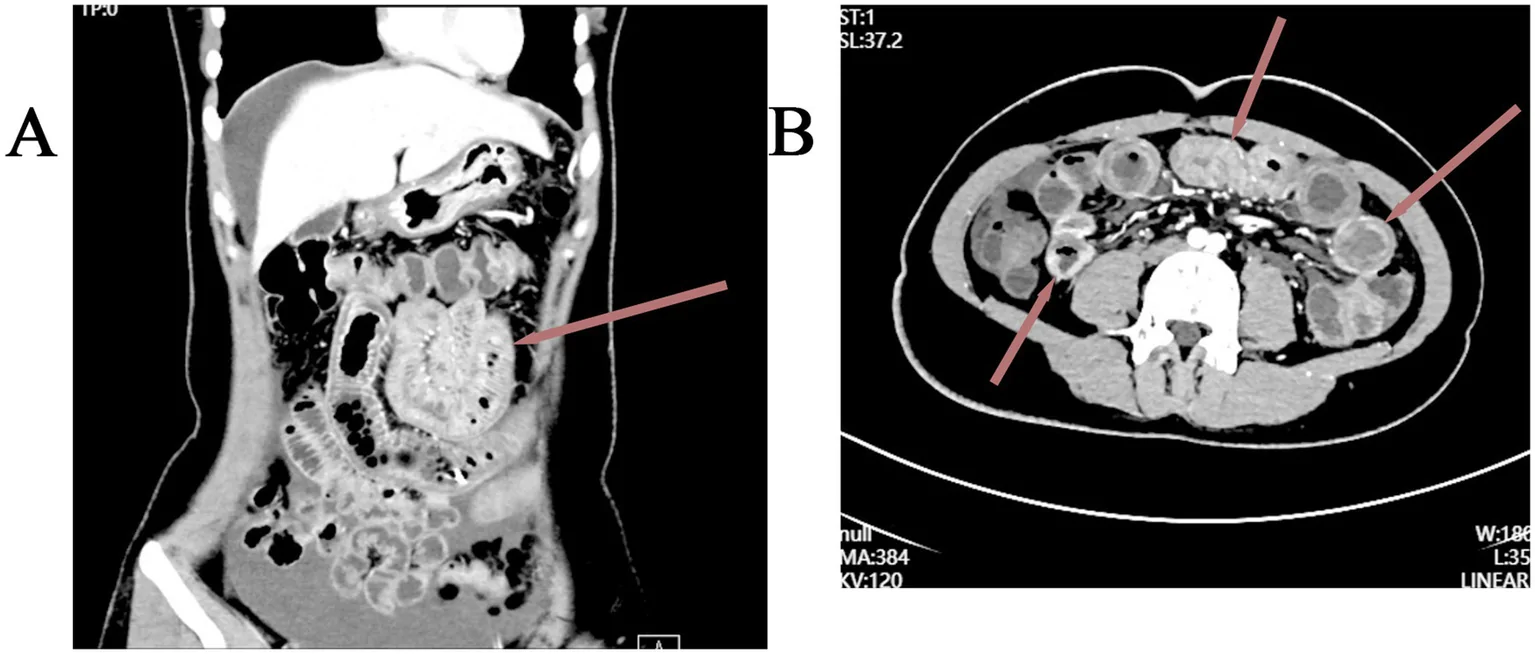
On September 3, 2024, during an exacerbation of abdominal pain, abdominal computed tomography angiography (CTA) revealed diffuse thickening and edema of the jejunal wall compared with previous scans, along with new-onset abdominopelvic fluid (A, B).
